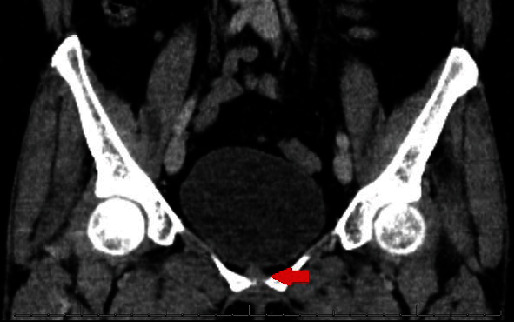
CT urogram with a focal thickening of the anterior bladder wall accompanied by minimal adjacent stranding.
